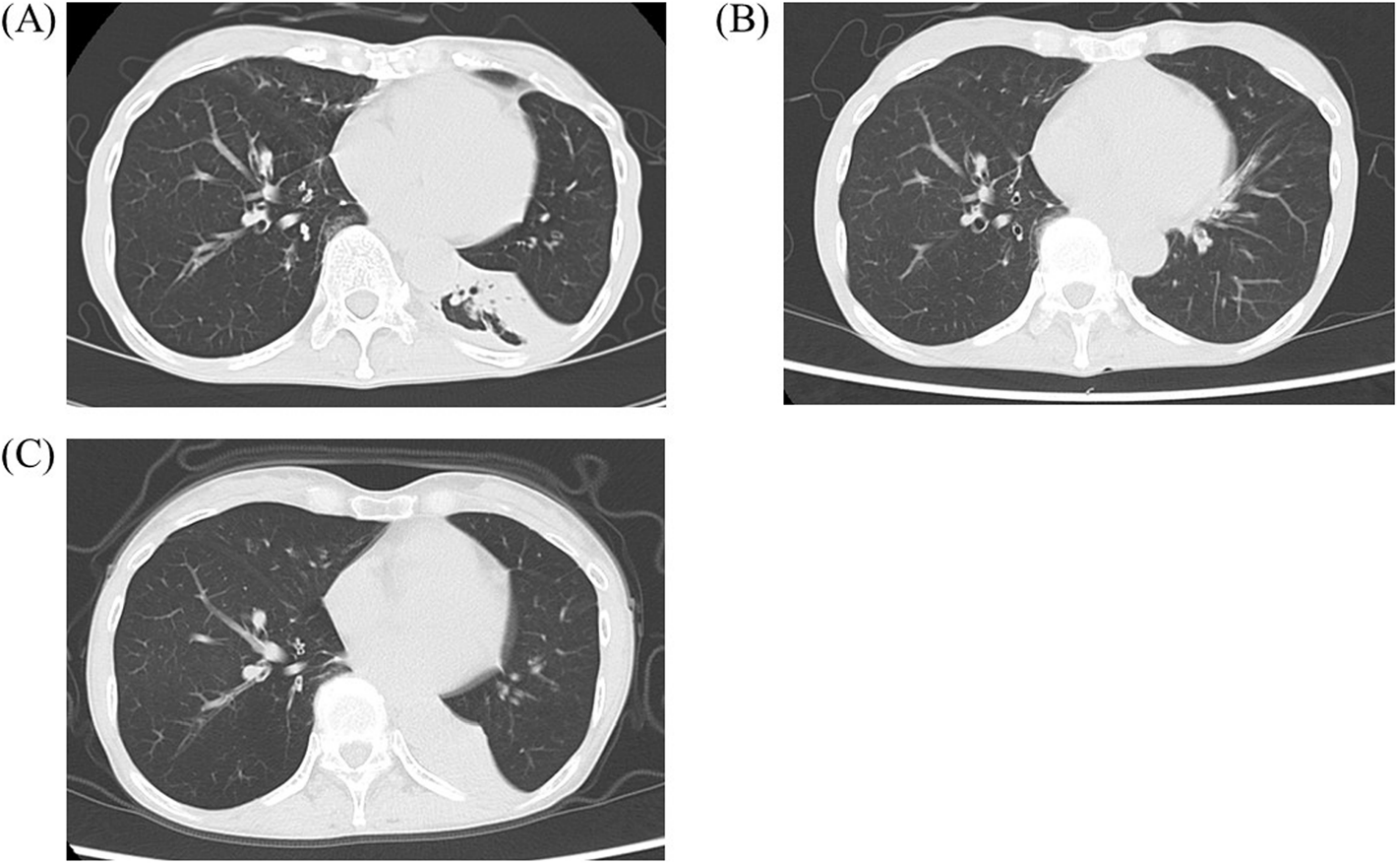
Chest computed tomography. a: Before treatment; b: 7 days after the initial treatment; and (c): 20 months after the initial treatment
